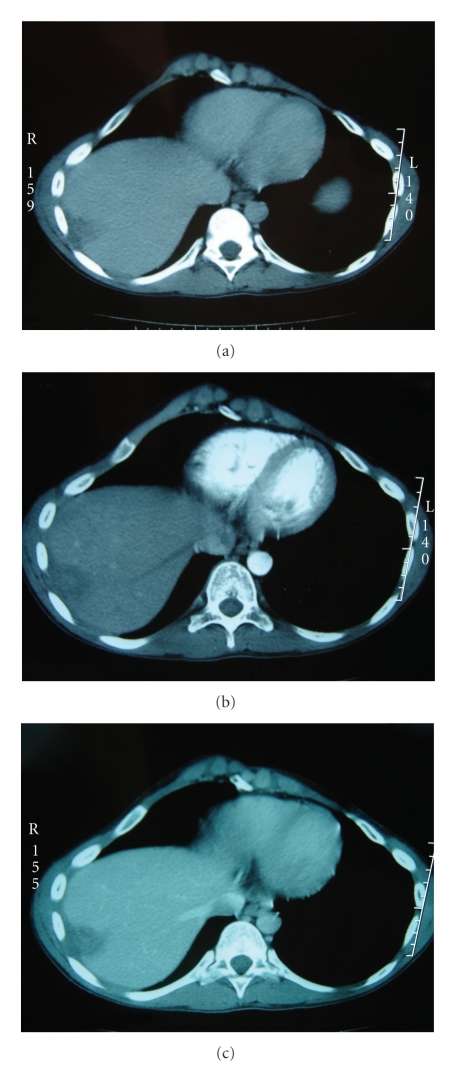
CT scan showing subcapsular lesion occupying segment VII and VIII and measuring 4 cm in diameter spontaneously low attenuated and having slight peripheral enhancement at delayed stage.
